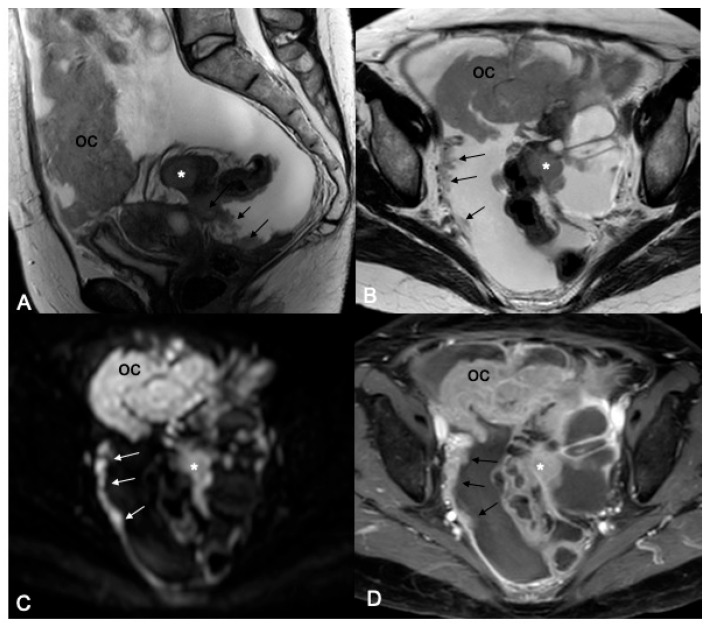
Sagittal (A) and axial (B–D) MR images of the pelvis. Sagittal (A) and axial (B) T2W images show a 3 cm peritoneal deposit infiltrating the sigmoid colon (asterisk). There is also involvement of the pelvic peritoneum and Douglas pouch (arrows). “Omental cake” also present (oc). Peritoneal disease demonstrates high signal intensity on the b 1200 DWI image (C), indicative of restricted diffusion, and enhancement on the T1W post-contrast sequence with fat saturation (D).
